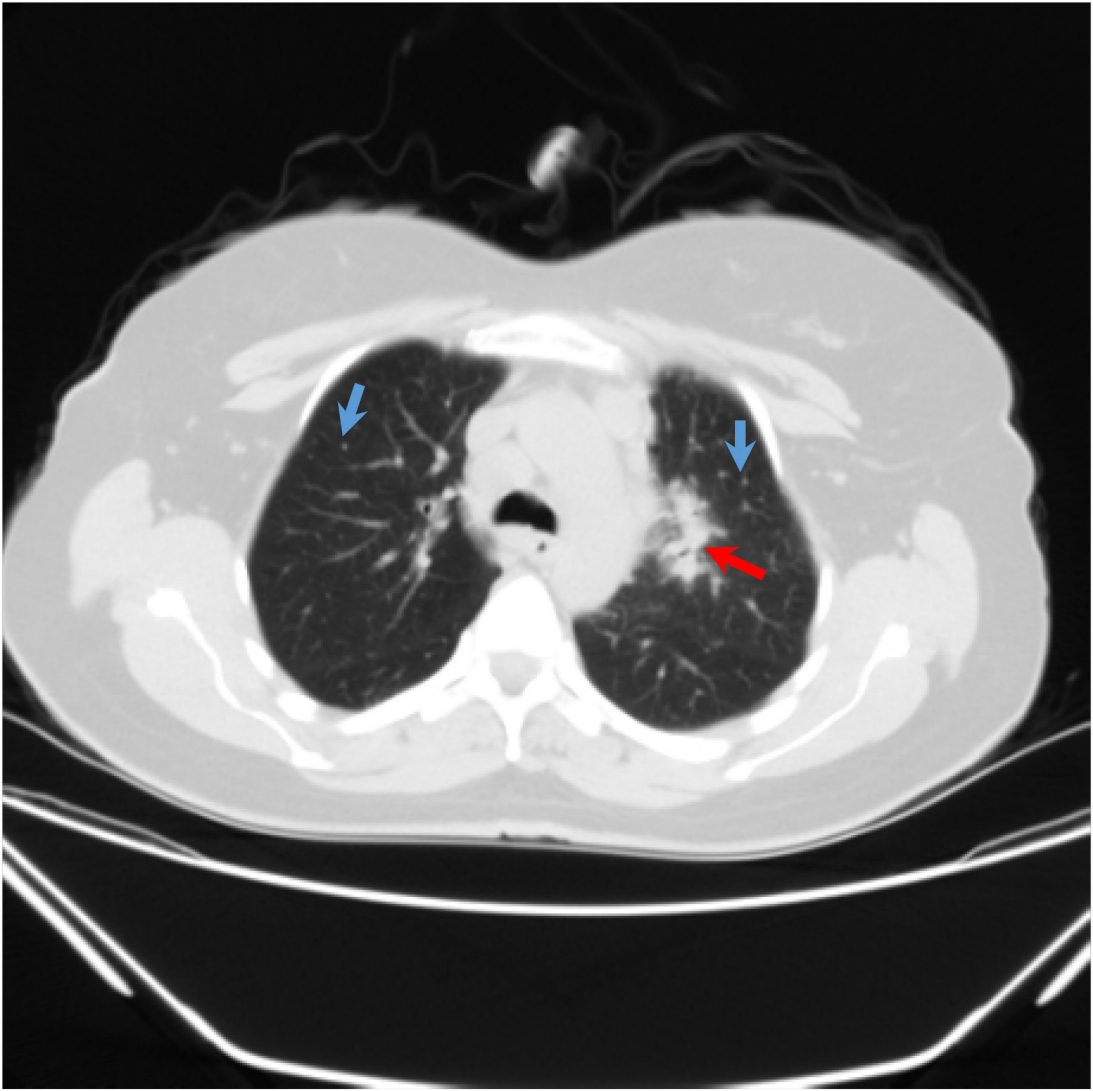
Lung HRCT, showing left hilar mass (red arrow) and bilateral nodules (blue arrows).
